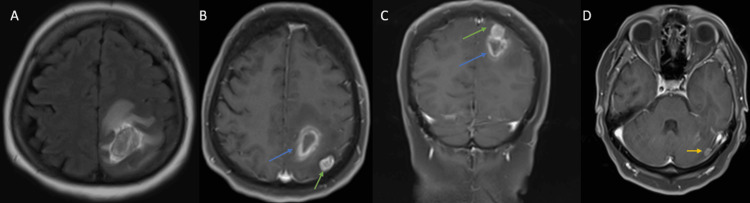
Brain MRI without and with IV contrast A: Axial flair; B, C, and D: coronal and axial T1 post-contrast images. There is the peripheral enhancement of the mentioned lesion (blue arrow); however, another nodular-enhancing lesion is seen post-contrast. One is located adjacent to the former in the left parietal lobe (green arrow), in addition to a smaller one in the left cerebellar hemisphere (yellow arrow).
